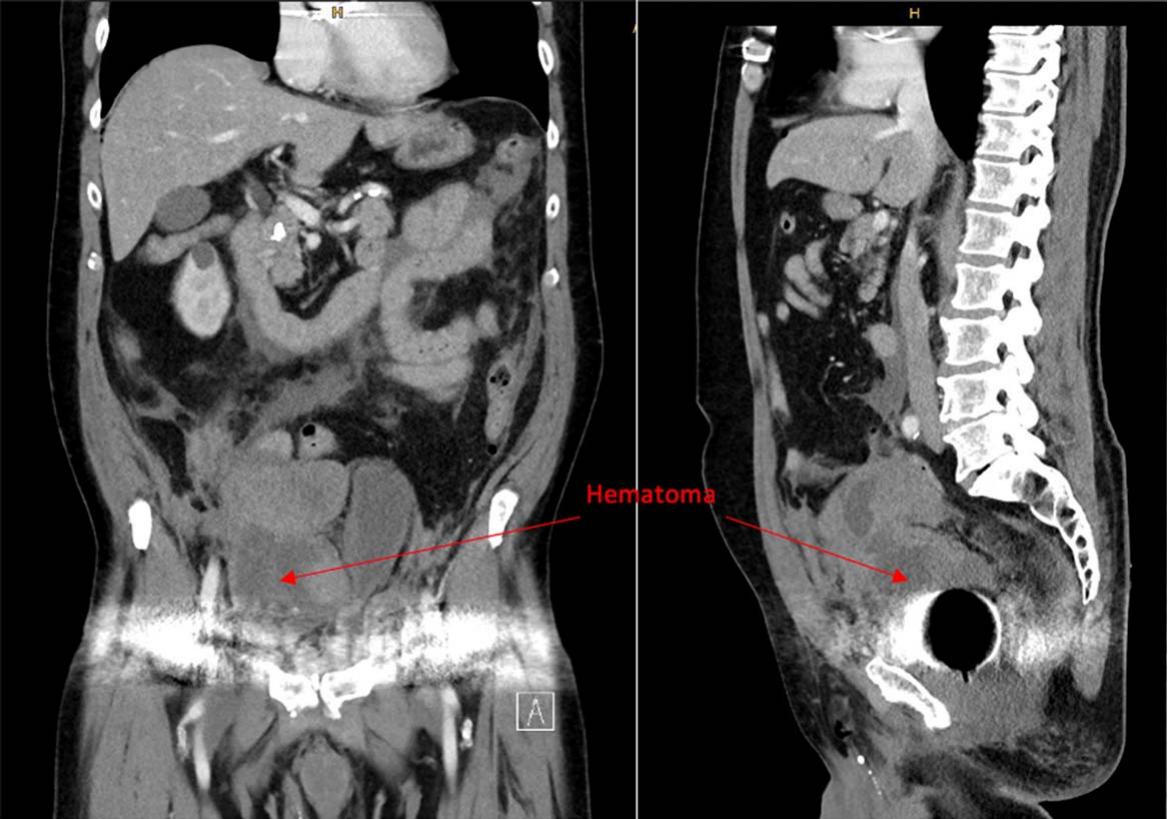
Computed tomography scan with supra-symphyseal hematoma left (arrow: hematoma).
